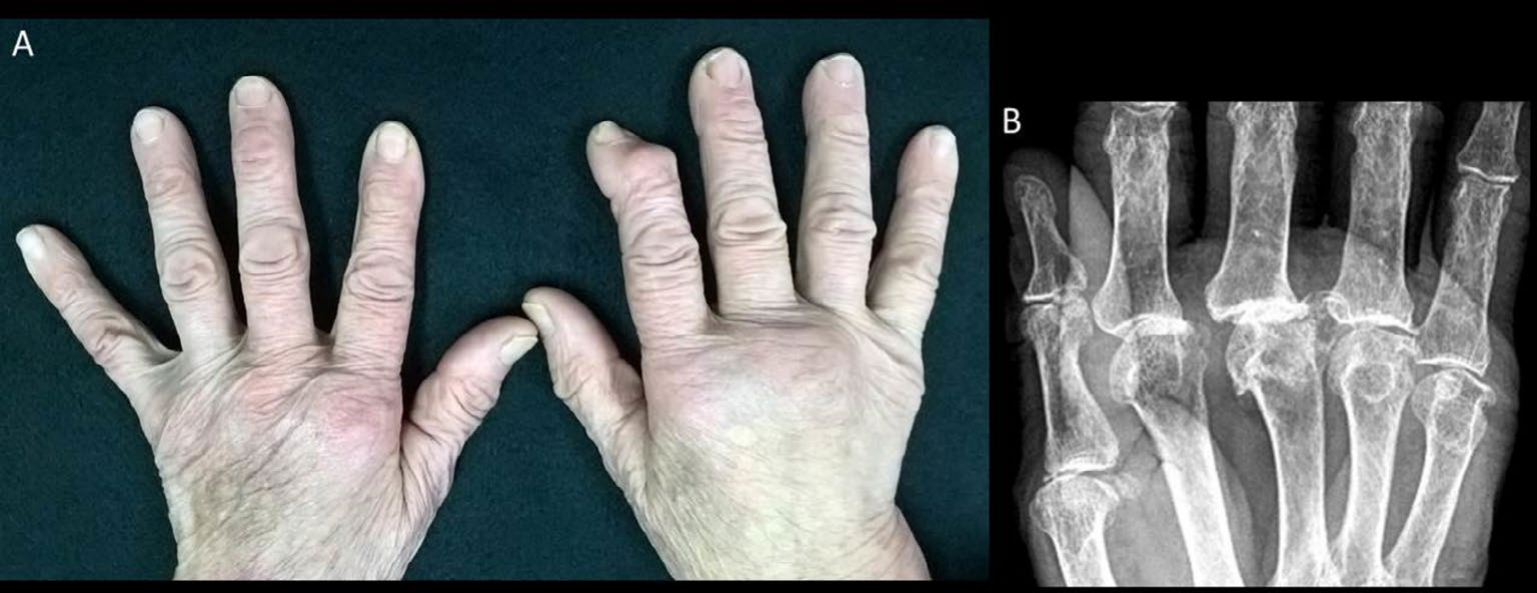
Hand osteoarthritis. A 79‐year‐old man presenting with predominantly mechanical pain and ‘hard’ swelling of the second and third metacarpophalangeal (MCP) joints of the right hand. The clinical picture, initially interpreted as seronegative rheumatoid arthritis, was refractory to multiple lines of treatment with both conventional synthetic and biologic disease‐modifying antirheumatic drugs. Subsequent re‐evaluation—including radiographic, clinical (noting the patient's occupational history as a plumber), and laboratory assessments—allowed for the exclusion of rheumatoid arthritis, supporting a diagnosis of hand osteoarthritis. (A) Clinical image showing swelling of the second and third right MCP joints. (B) Radiographic detail of the right metacarpal region demonstrating osteoarthritic changes (marked joint space narrowing, particularly at the third MCP joint, osteophyte formation, and subchondral sclerosis).
